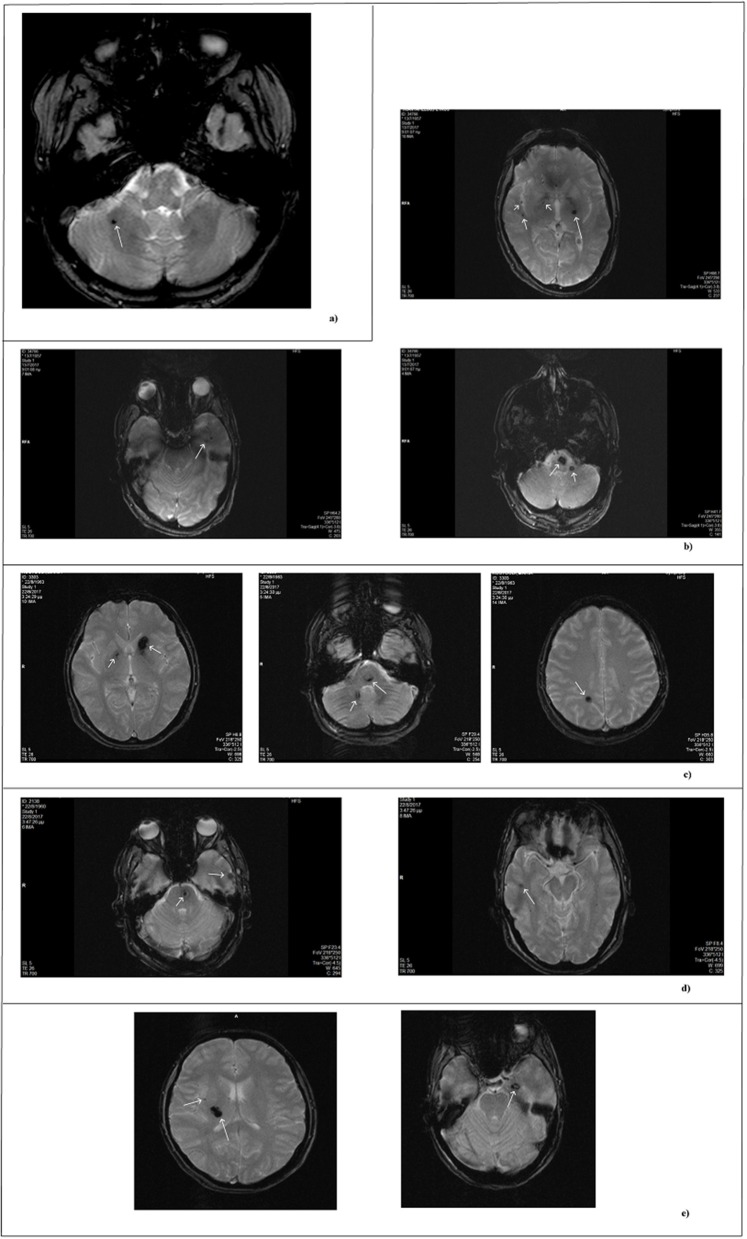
MRI scan of patients. MRI images obtained by T2- weighted gradient echo sequences. Each panel refers to a single patient. (a) Single lesion detected in the sporadic patient. (b–e) Lesions of affected family members. In reference to Figure 2: (b) II-1; (c) II-3; (d) II-4; (e) III-13.
